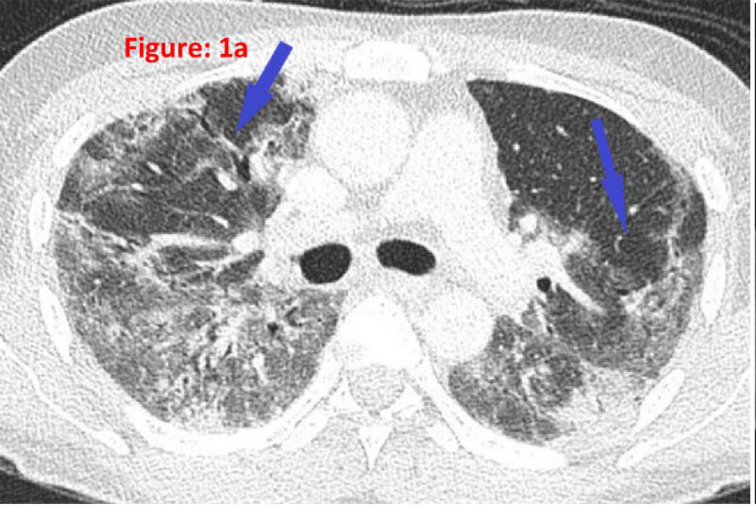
High-resolution chest computed tomography (HRCT) showing extensive ground-glass opacities at the peripheral and subpleural regions with fibrotic changes (blue arrows).
